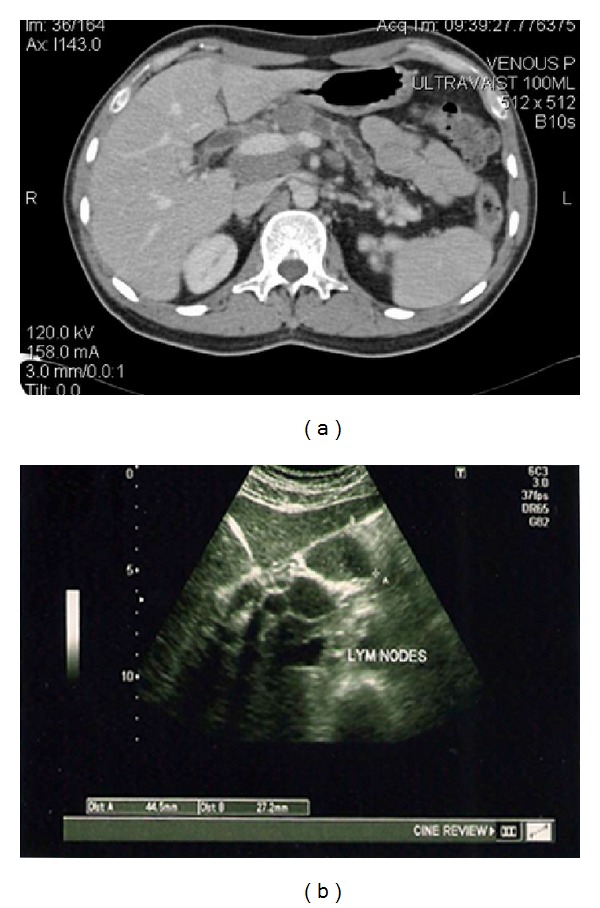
18 months after initial presentation. (a) CT showing persistent enlarged peripancreatic lymph nodes, pancreatic atrophy, and dilatation of pancreatic duct. (b) Ultrasound abdomen showing enlarged peripancreatic lymph nodes.
